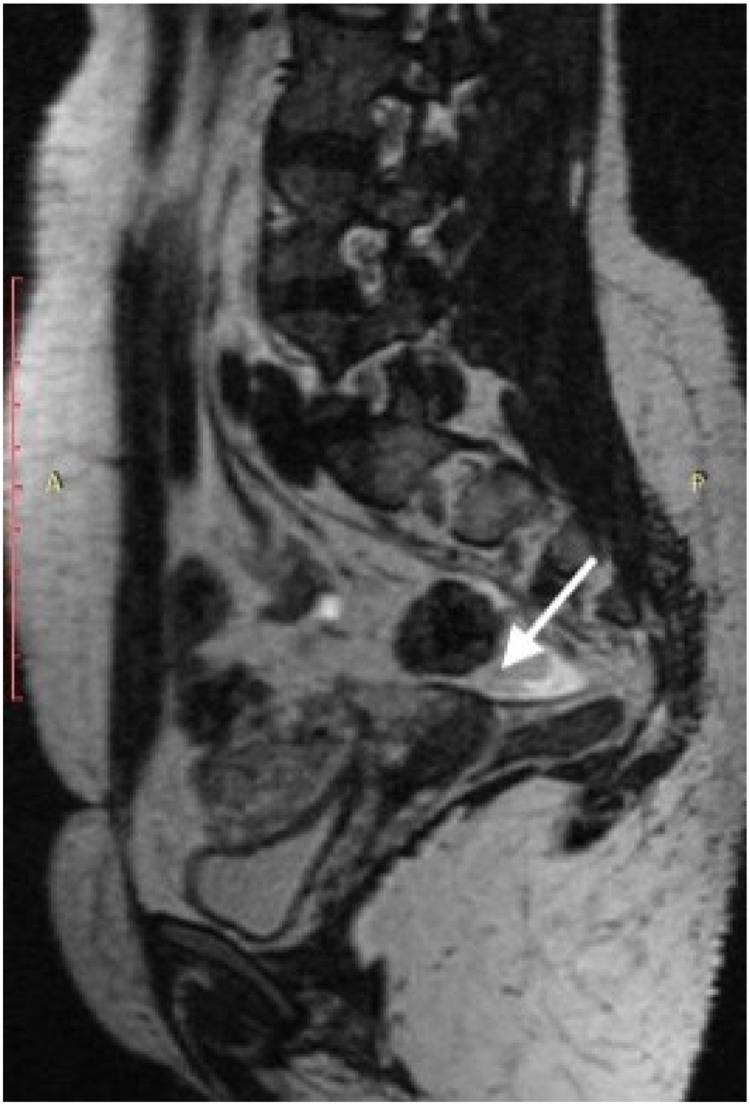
Sagittal T2-weighted pelvic magnetic resonance image showing a low-signal-intensity thickening in the retrocervical region, extending to the left paracervical area and left uterosacral ligament (arrow), consistent with deep infiltrating endometriosis.
